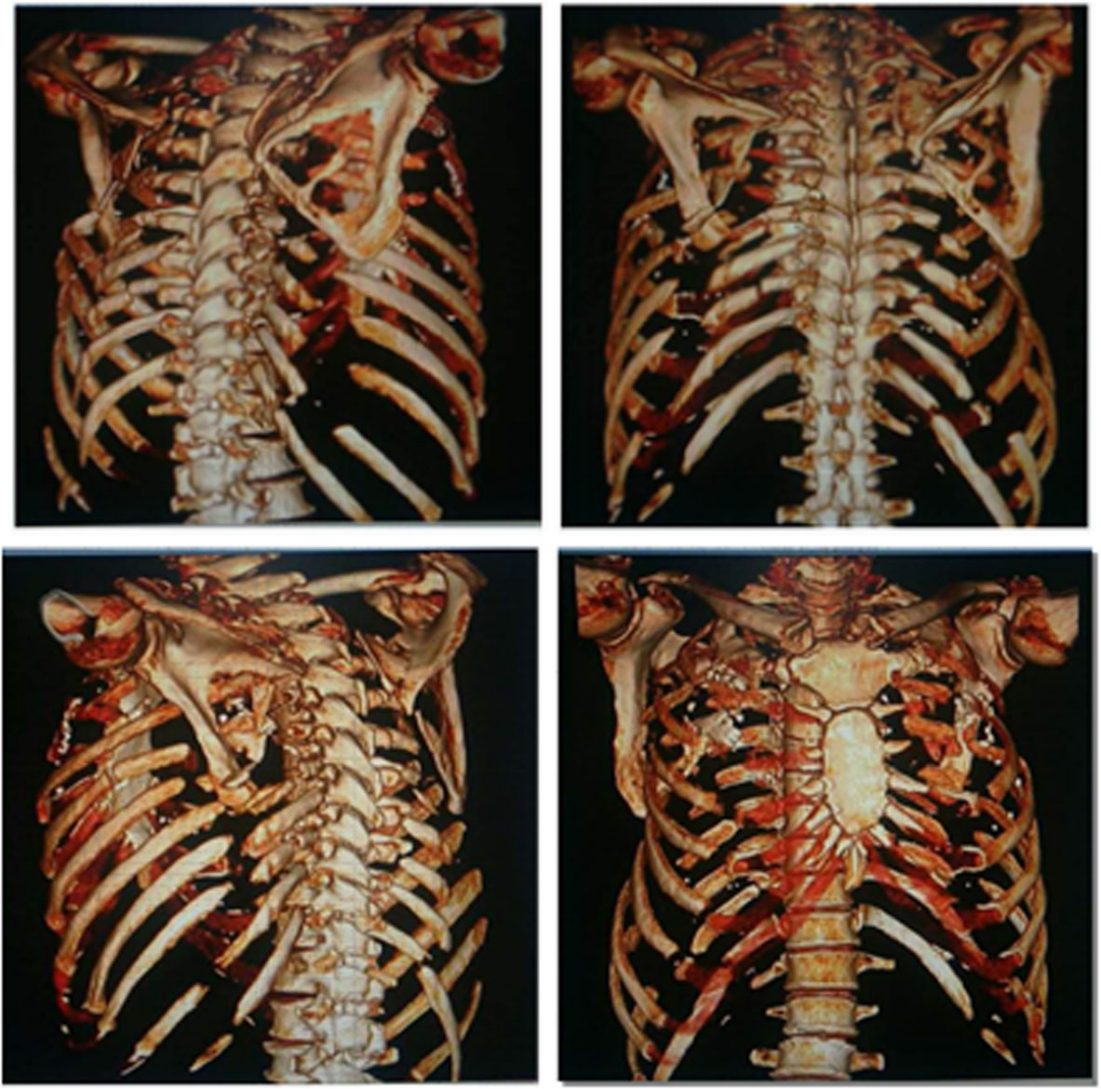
Chest CT (3D reconstruction): bilateral anterior and posterior multiple rib fractures (2nd-10th on the right side and 2nd-11th on the left side)
